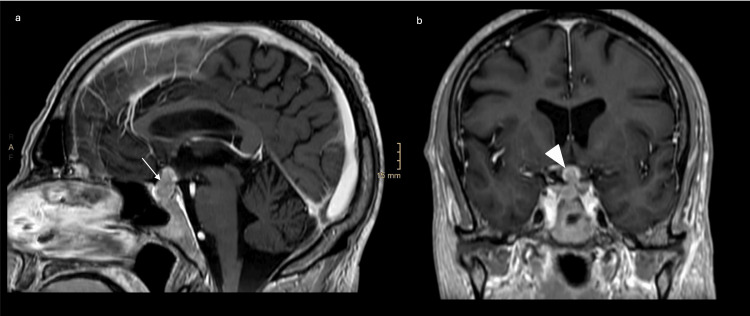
Initial cranial MRI with contrast medium (a) Sagittal T1-weighted MRI showing a contrast-enhancing mass in the pituitary fossa (arrow) and (b) a coronal T1-weighted MRI showing a contrast-enhancing mass (arrowhead) without infiltration of the cavernous sinus at the patient's initial presentation.
